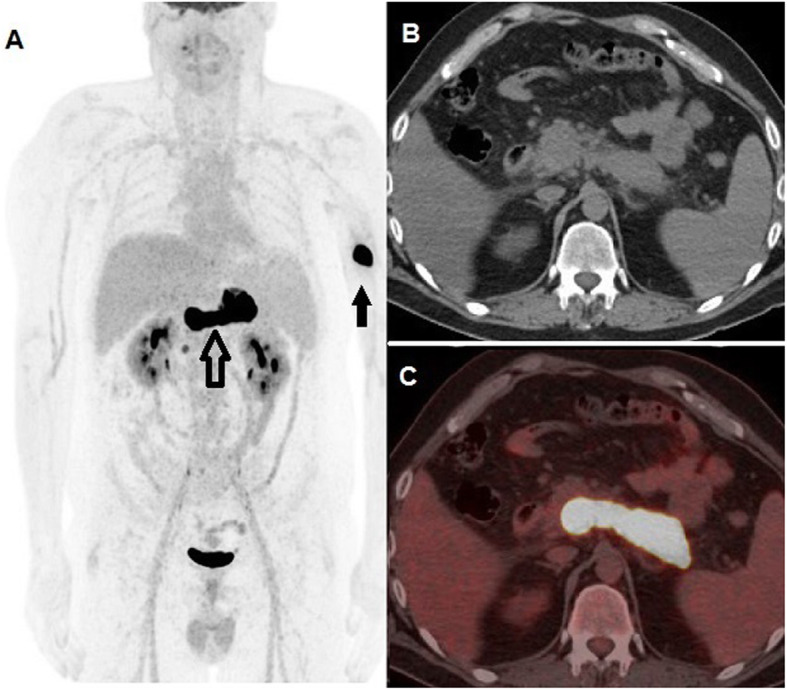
Whole body ^18^F-FDG PET/CT (A) demonstrated extremely increased tracer incorporation in the cutaneous/subcutaneous metastasis in the left arm (black arrow) with maximum standardized uptake value (SUVmax) of 67.3 and diffuse and intense ^18^F-FDG uptake in the whole pancreas (SUVmax 99.3, black bordered arrow), which resulted significantly enlarged at the corresponding CT (B) and fused PET/CT (C) axial slices
